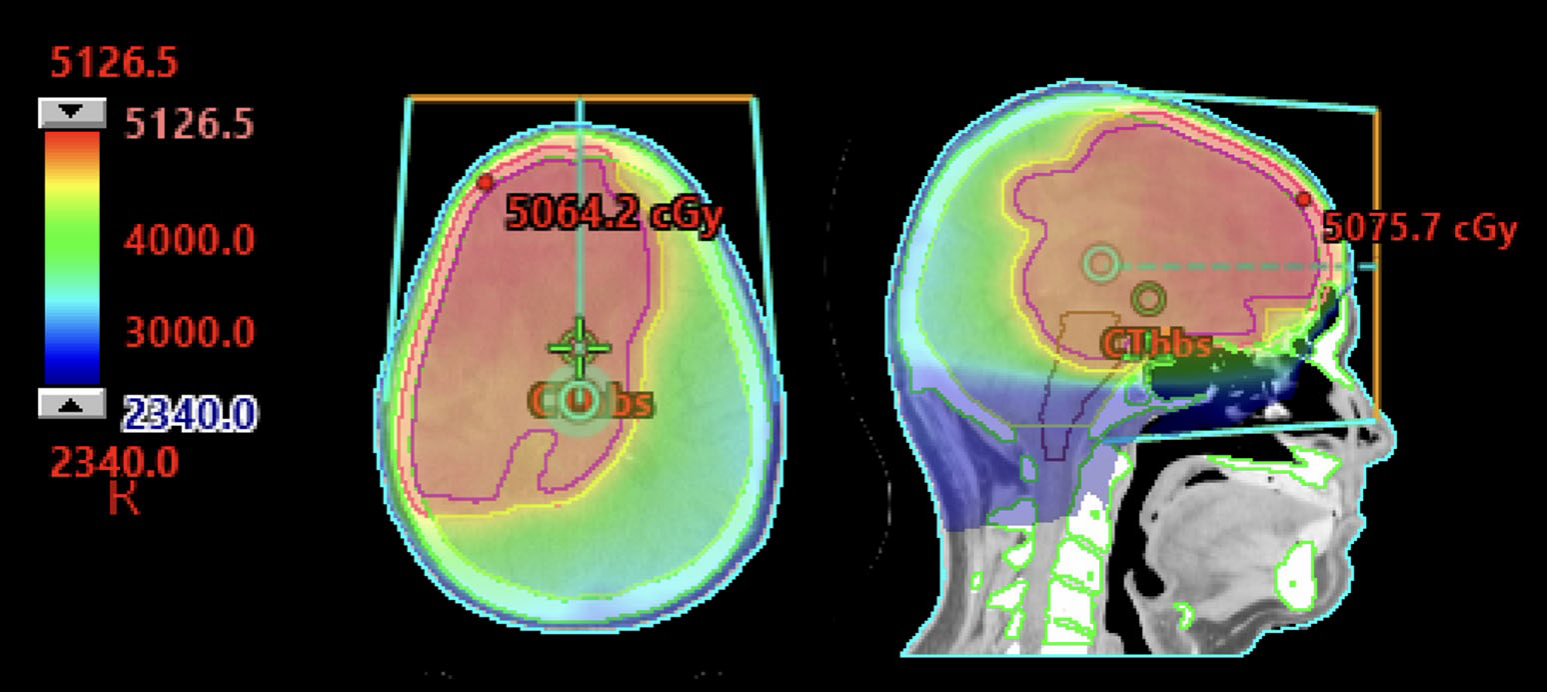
63-year-old immunocompetent man with primary CNS lymphoma treated per CALGB 50202 protocol with methotrexate, temozolomide, and rituximab with partial response followed by autologous hematopoietic cell transplant. He had an early recurrence 4 months later with negative lumbar puncture and PET/CT. He was treated with 23.4 Gy in 13 fractions to the whole brain with a sequential boost to a total of 45 Gy in 25 fractions.
